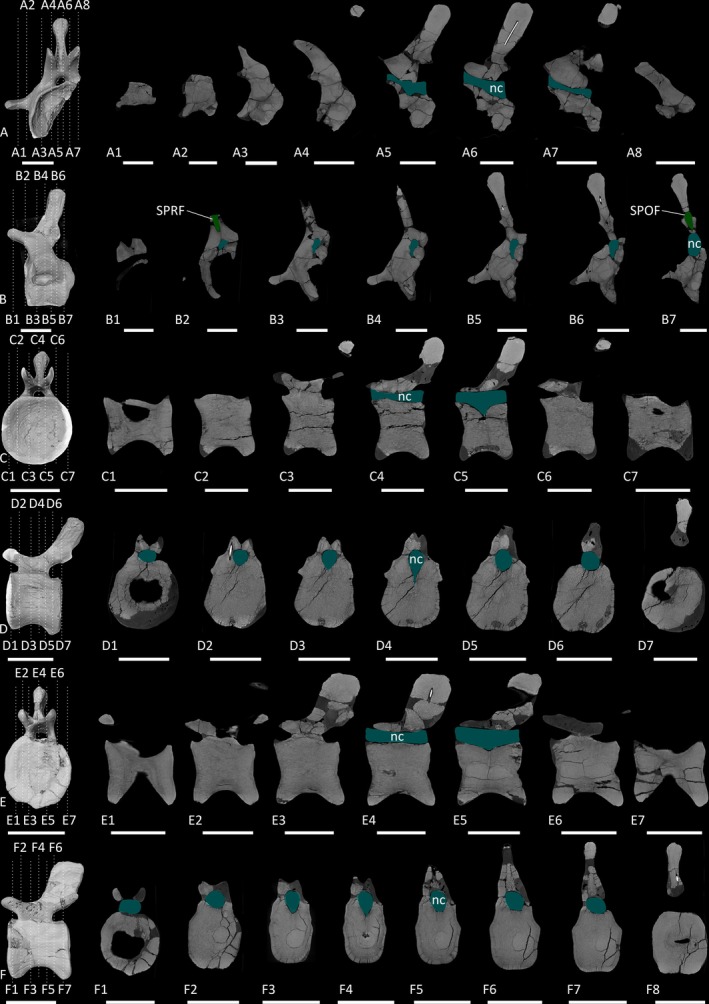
CT scan of *Wamweracaudia keranjei* anterior and middle caudal vertebrae. (A–F) 3D rendering (A, C, E) anterior view, (B, D, F) left lateral view; (A, B) anterior caudal vertebra MB.R. 2091.27, (C, D), middle caudal vertebra MB.R. 2091.19, (E, F) middle caudal vertebra MB.R. 2091.16; (A1–A8, C1–C7, E1–E7) CT scan slices in parasagittal cross‐section, (B1–B7, D1–D7, F1–F8) CT scan slices in transverse cross‐section. nc, neural canal; SPOF, spinopostzygapophyseal fossa; SPRF, spinoprezygapophyseal fossa. Key: Blue highlight, neural canal; green highlight, neural spine fossa. Scale bars = 100 mm.
